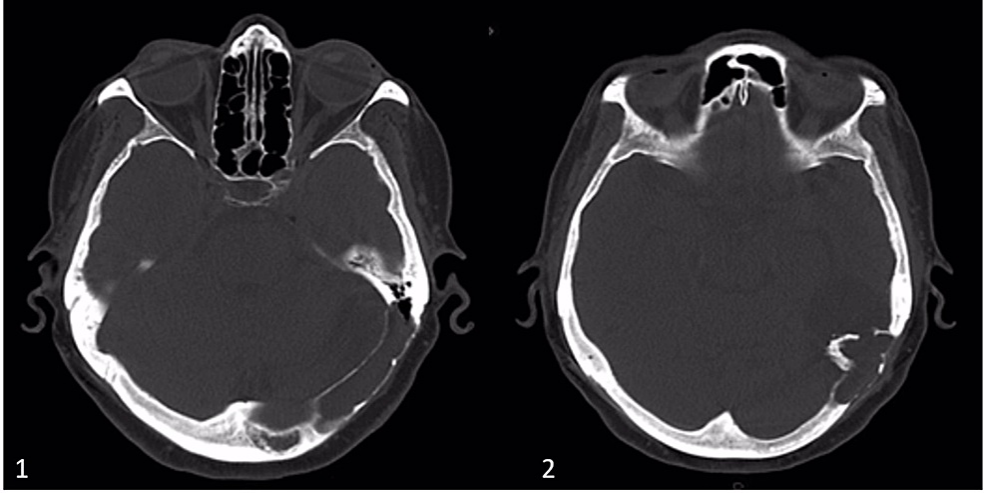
Preoperative axial CT scans showing lesions in the diploe of petrous and occipital bones.
